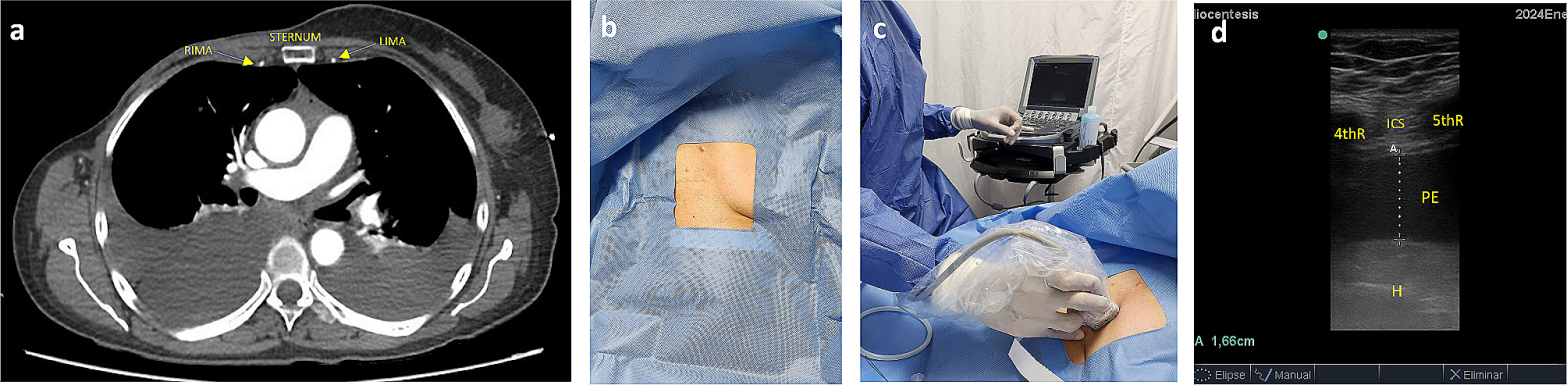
(a) CT scan showing LIMA and RIMA being laterally around 1.5 mm from the sternum. CT: computed tomography, RIMA: right internal mammary artery, LIMA: left internal mammary artery (b) Asepsis and antisepsis before the procedure (c) Linear transducer in longitudinal plane (d) High frequency linear transducer showing the anterior pericardial effusion. ICS: inter costal space, PE: pericardial effusion, H: heart, 4thR: 4th rib, 5thR: 5th rib
